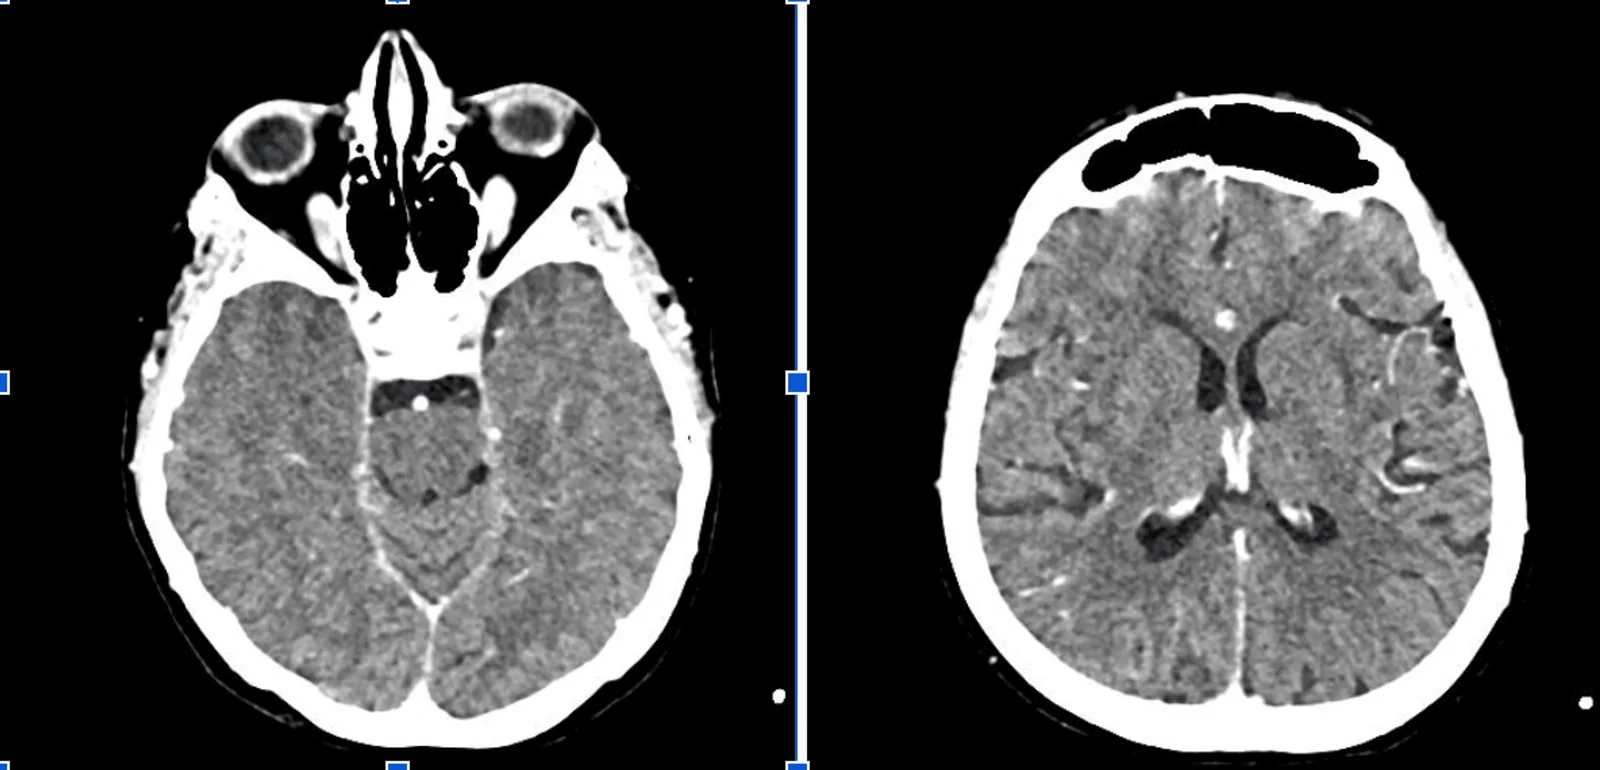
Cerebral CT angiography showing no arterial abnormality and no pathological parenchymal enhancement in the supratentorial region
